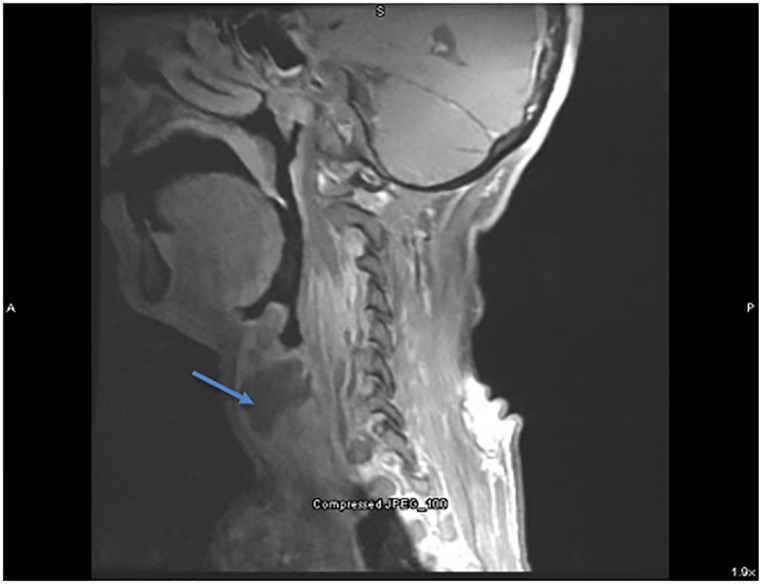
Magnetic resonance imaging of the neck (sagittal view) showing anterior fluid collection partially obstructing the trachea (blue arrow).
